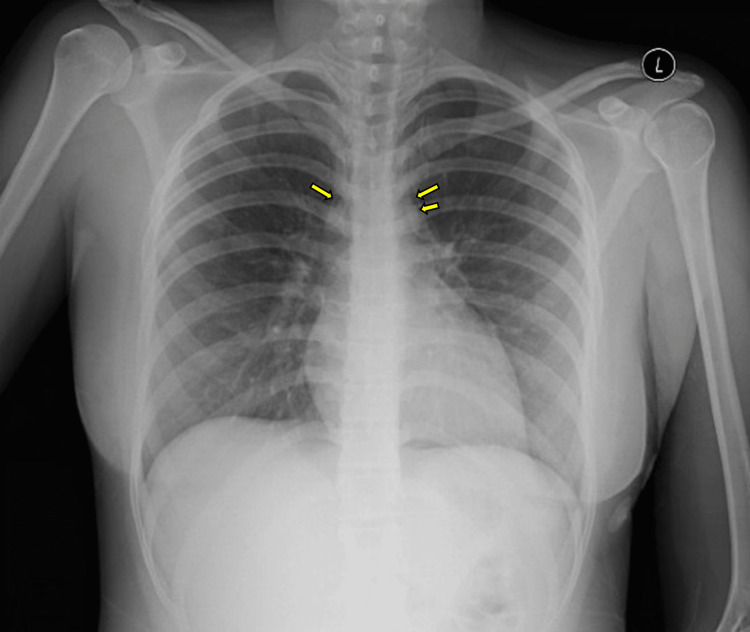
Chest radiograph of a PM Posteroanterior chest radiograph showing probable PM with clear lungs and no pleural effusion or pneumothorax. Arrows denote lucent streaks of extraluminal air in the mediastinum PM: pneumomediastinum
